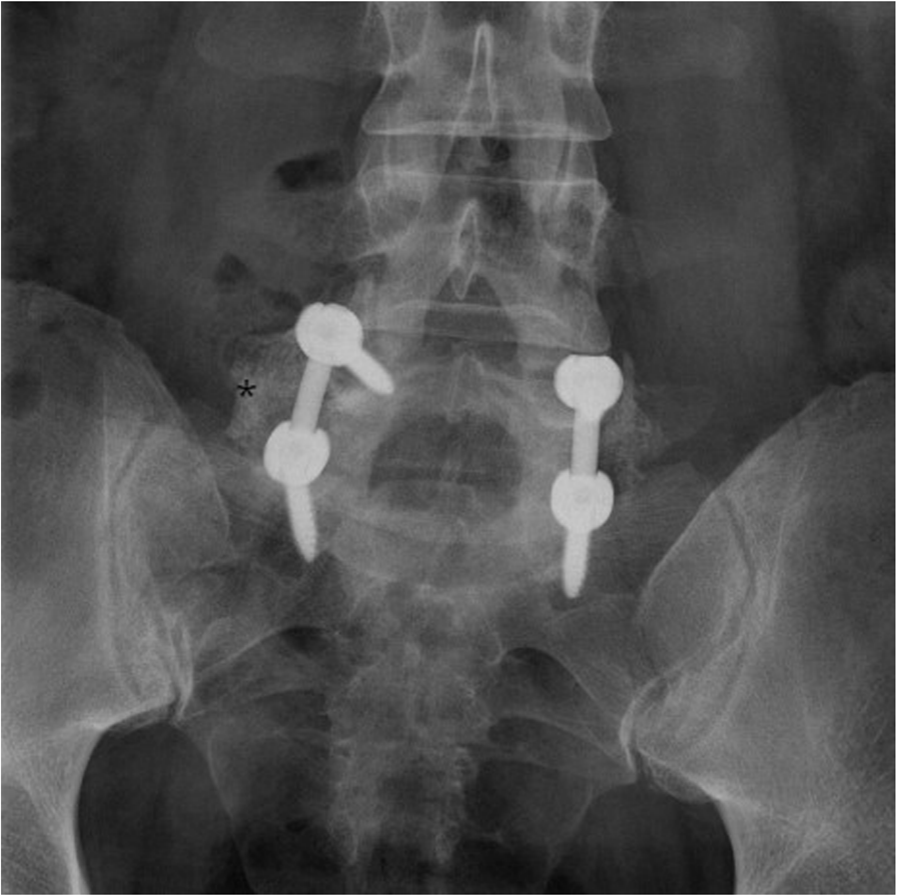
Anteroposterior X-ray of L5–S1 showing bone bridges (asterisk) formation in the intertransverse space for posterolateral spine fusion 1 year after surgery
